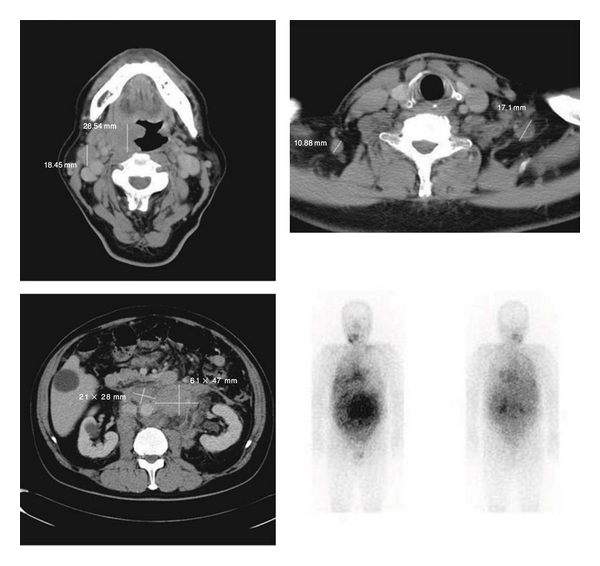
Computed tomographic scan (CT) showing the swelling of the right tonsil and the enlargement of right cervical, left supraclavian, and para-aortic lymph nodes. 67 Ga scintigraphy showing intense uptake in the same lesions which were detected in CT.
